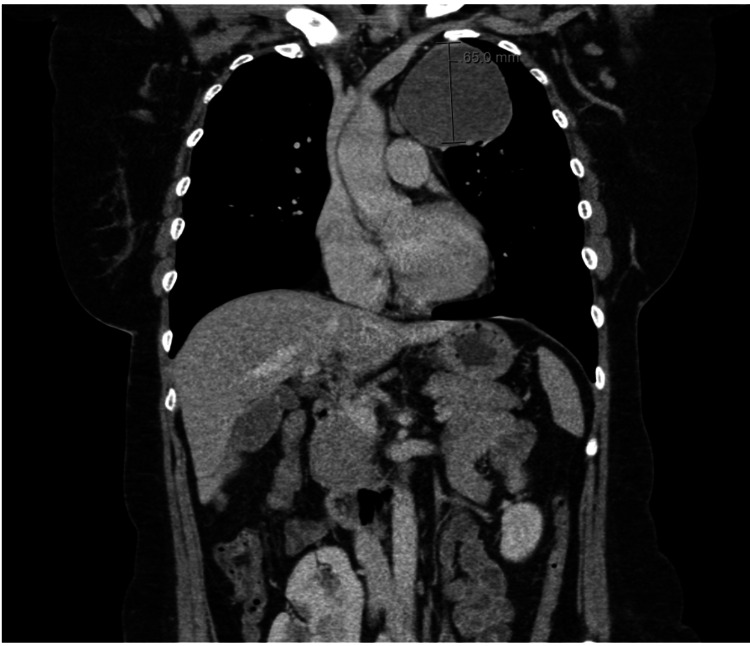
Coronal view contrast-enhanced CT thorax showing a well-defined anterior mediastinal hamartoma
